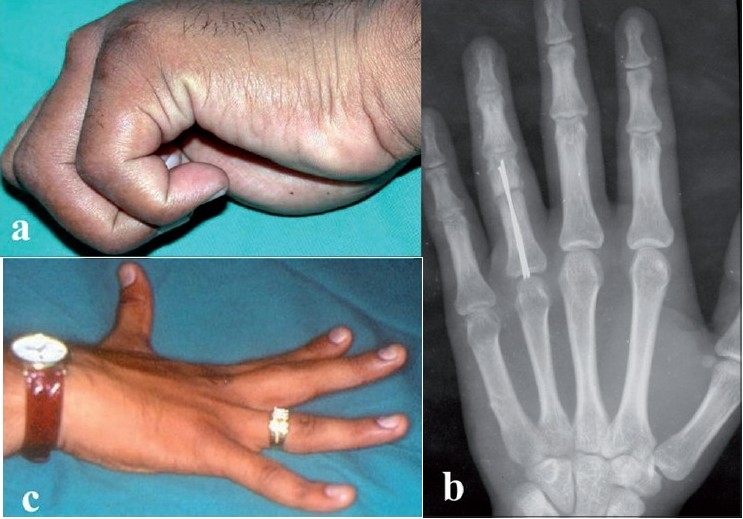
(a) Clinical photograph of adventitious bursitis at the site of entry portal in one patient (b) X-ray depecting of backing of nails causing adventitious bursitis. (c) Clinical photograph of 5° extension lag at proximal interphalangeal joint in one of the patients
